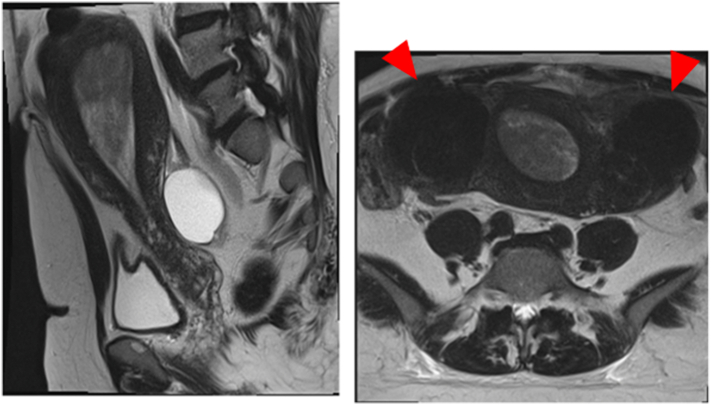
Sagittal T2-weighted MRI: Uterus in a neutral position with a 4-cm endometrial thickening and a 6-cm bifurcated cyst in the left ovary (left). Axial T2-weighted MRI: huge leiomyomas on both sides of the uterine fundus (arrowheads) (right). Abbreviations: MRI, Magnetic resonance imaging.
